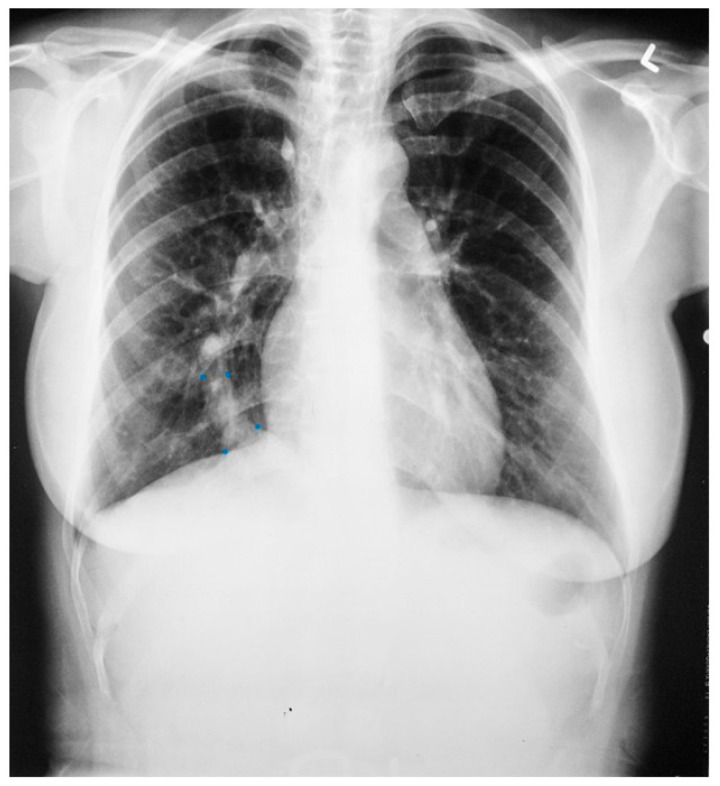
Posteroanterior chest X-ray of Scimitar syndrome: This is a 40-year-old asymptomatic female who had a chest X-ray as part of a routine physical examination. The film is well centered and there is a good inspiration. The ratio is normal. The right lung is normal in size, but note at the right lung apex there is an azygos fissure with a laterally-displaced azygos vein. There is a discrete scimitar paralleling the right heart border. Notice how the caliber of the scimitar increases as it courses inferiorly. The right-to-left distance between the upper two blue dots that straddle the scimitar is smaller than the distance between the lower two scimitar-straddling blue dots. The fact that the pulmonary arteries are not plethoric indicates that the left-to-right shunt (right lower pulmonary vein to inferior vena cava) is not large. Cardiac auscultation corroborated this. There was no systolic pulmonary flow murmur and S2 demonstrated physiological splitting that was not widely split or fixed. There was mild right ventricular volume overload by echocardiography. Her exercise capacity was normal. She received no treatment and periodic F/U was scheduled.
